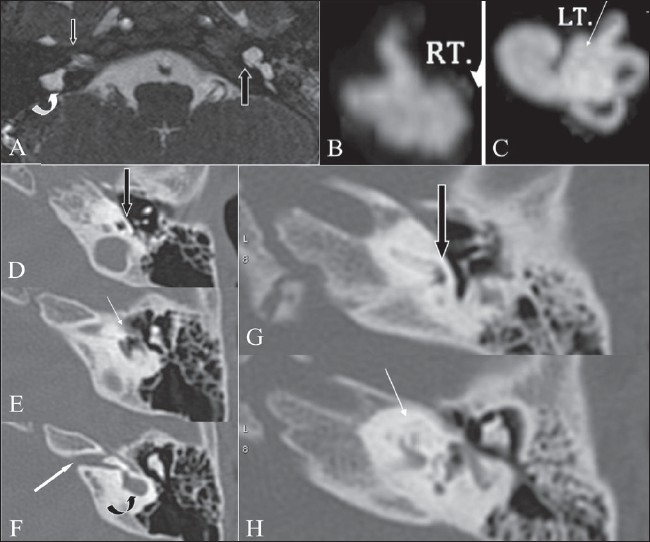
Cochlear aplasia and Incomplete partition type I deformity. Axial 3D-FIESTA image (A) shows an absent right cochlea, suggesting cochlear aplasia (arrow), along with a deformed / dysplastic vestibule (curved arrow). On the left side, a dilated cystic cochlea is noted, with complete lack of the modiolus. 3D-MIP reconstruction images (B, C) of the image data set of Figure 4A, clearly show an absent cochlea on the right (B). On the left (C), a dilated cochlea is noted, with a cystic vestibule, consistent with an incomplete partition type I deformity. Axial HRCT images (D-F) at three consecutive levels show cochlear aplasia (white arrow in E), with loss of the cochlear promontory bulge (block black arrow in D) normally produced by the basal turn of cochlea (compare with Figs. 4G and 4H). At a higher level (F), a small IAC is noted (white arrow) along with a dysplastic cystic vestibule (curved arrow). Axial HRCT images (G, H) of another patient with labyrinthitis ossificans (taken for comparison with Figs 4D, 4E and 4F), show complete ossification of the cochlea (white arrow in H). Note that the normal cochlear promontory bulge (block arrow in G) is maintained
